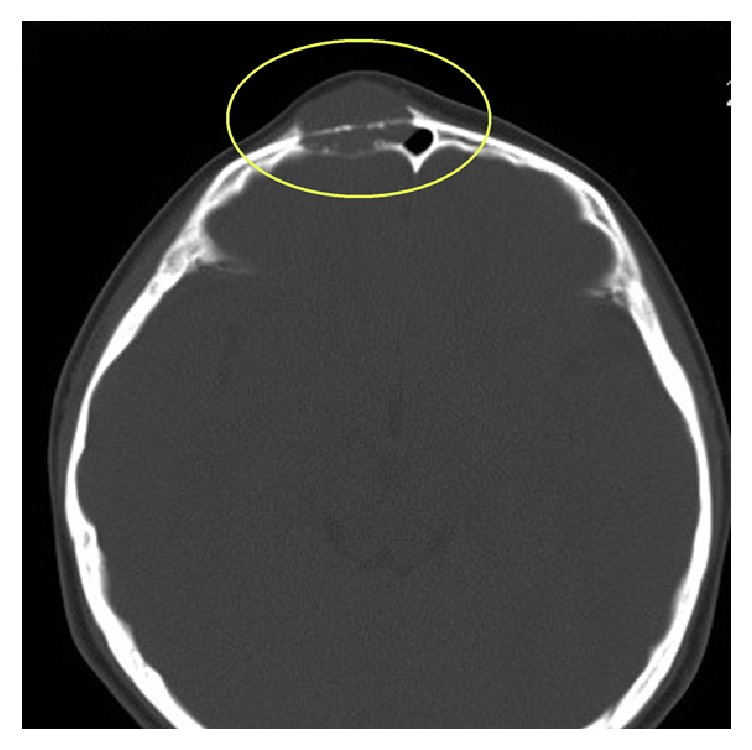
Images obtained from a 10-year-old female with IMT of the frontal bone. The CT scan showed destruction of the inner and outer plates, clear and sharp boundaries, and no calcification or ossification.
